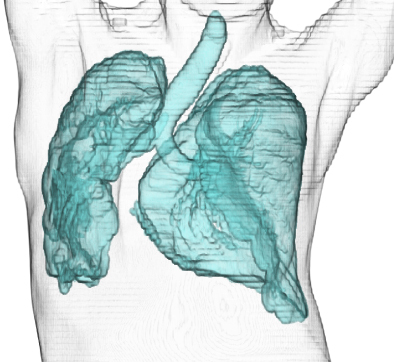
Three-dimensional chest computed tomography image showing the entire lung structure and reduction in lung volumes.
